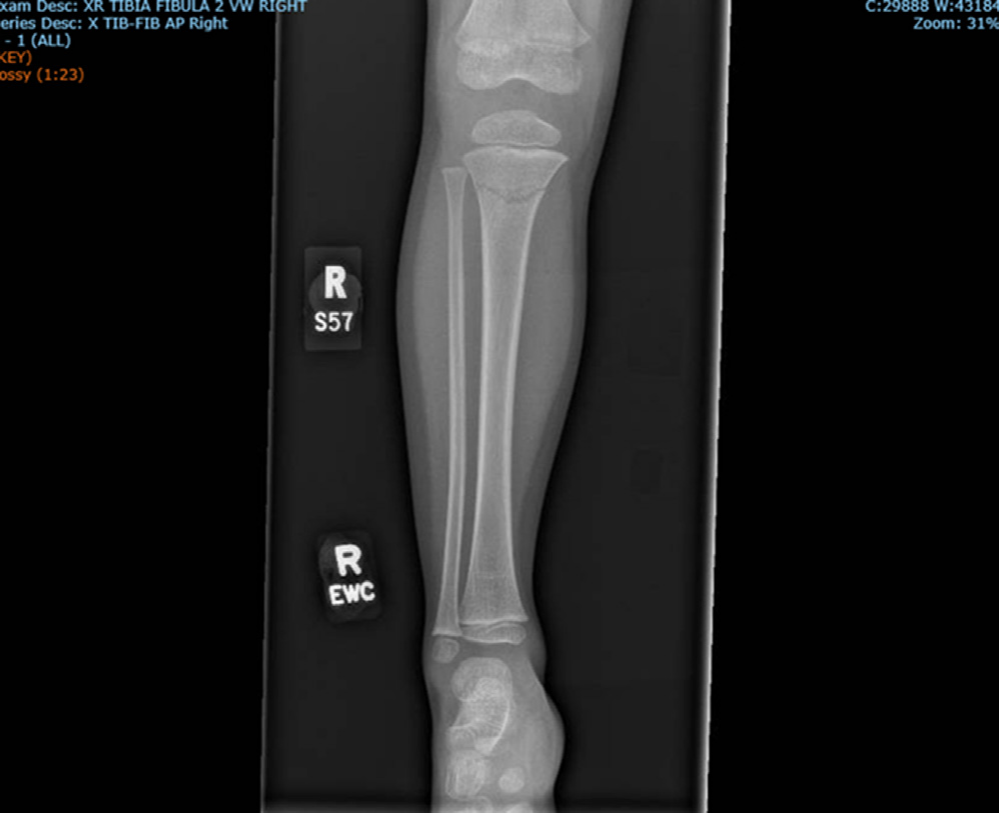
Anteroposterior plain film of right lower limb.
